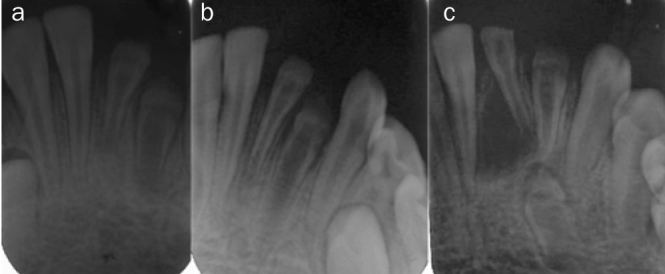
Treatment of patient F, followed for 13 years (from 7 to 24 years of age). Teeth 31, 32, and 33 are affected by RO. a) to c) Radiographs taken at age 9, 10 and 12 visualise maturation and a slow eruption of 31, 32 and 33 and development of a radicular cyst.
